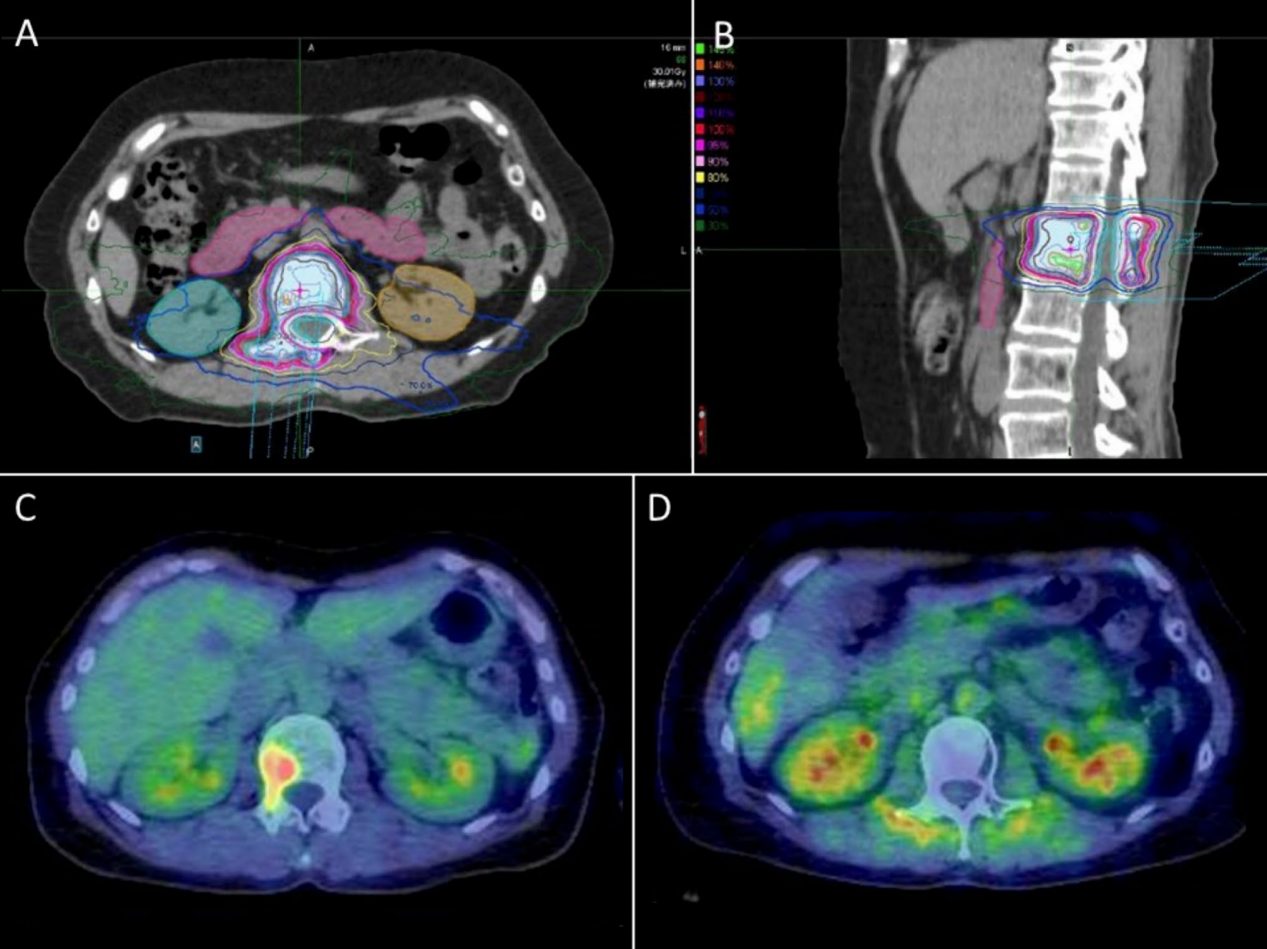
Radiation field and dose distribution of SBRT for oligometastatic bone disease. The dose gradient is made very steep, allowing for a high dose of 24 Gy in 2 fractions while reducing the dose to the spinal cord (A and B). The accumulation seen on FDG-PET before SBRT (C) disappeared at 6 months after SBRT (D). *SBRT* stereotactic body radiation therapy; *FDG-PET* fluorodeoxyglucose positron emission tomography
